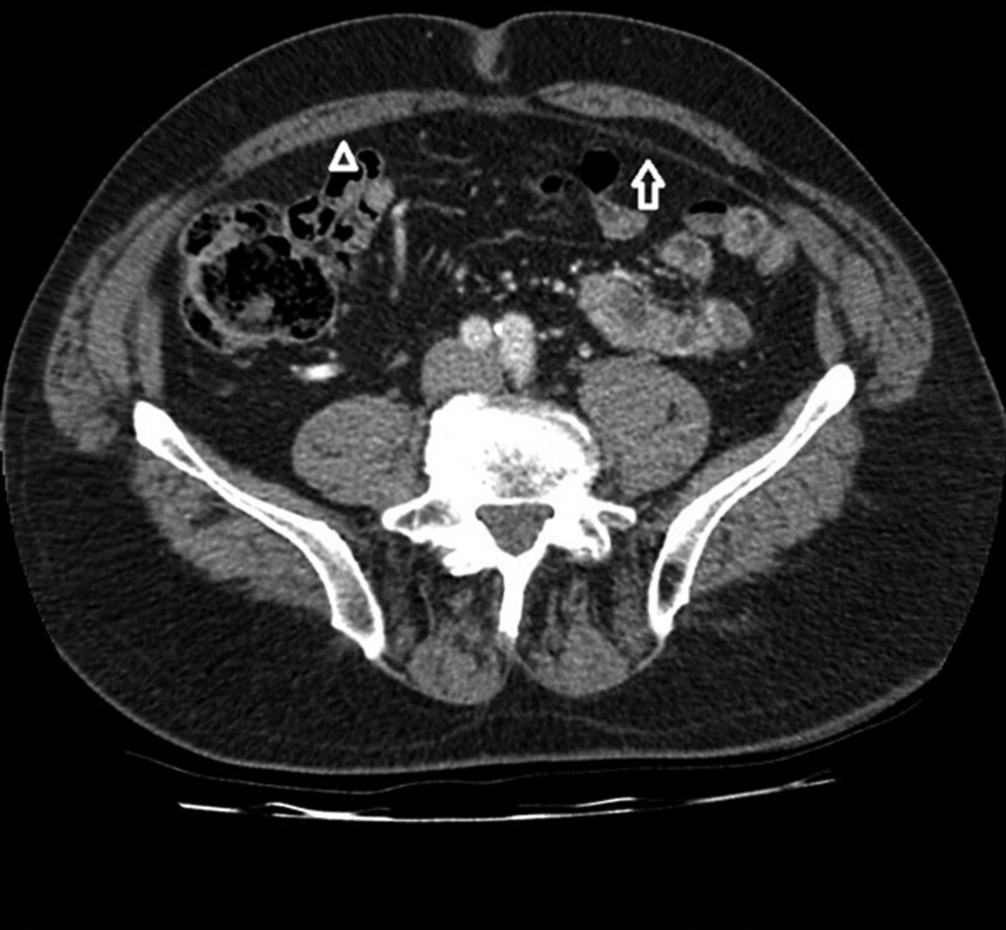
A contrast-enhanced, portal venous phase axial CT image shows splitting of the posterior sheath on the left side (white arrow) with intact sheath on the right side (arrowhead).
